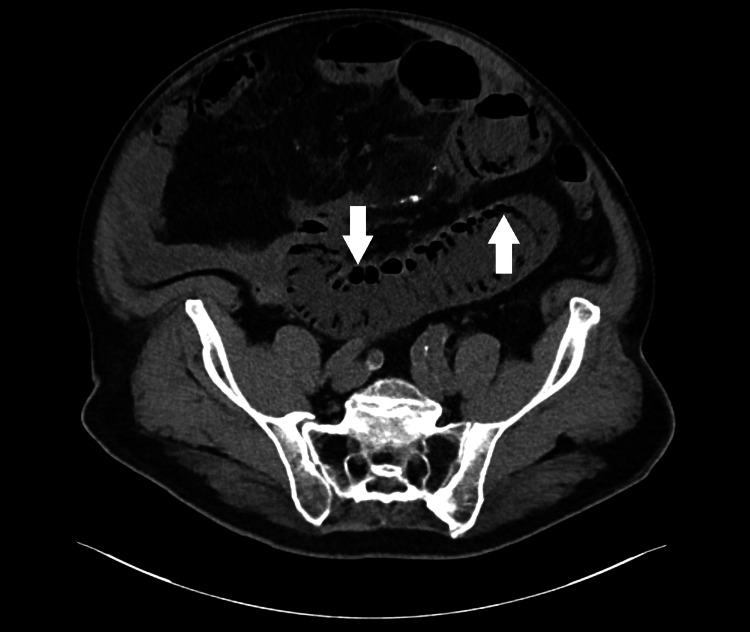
CT abdomen demonstrating pneumatosis intestinalis Hypodense air within the walls of the bowel is highlighted by the arrows.
